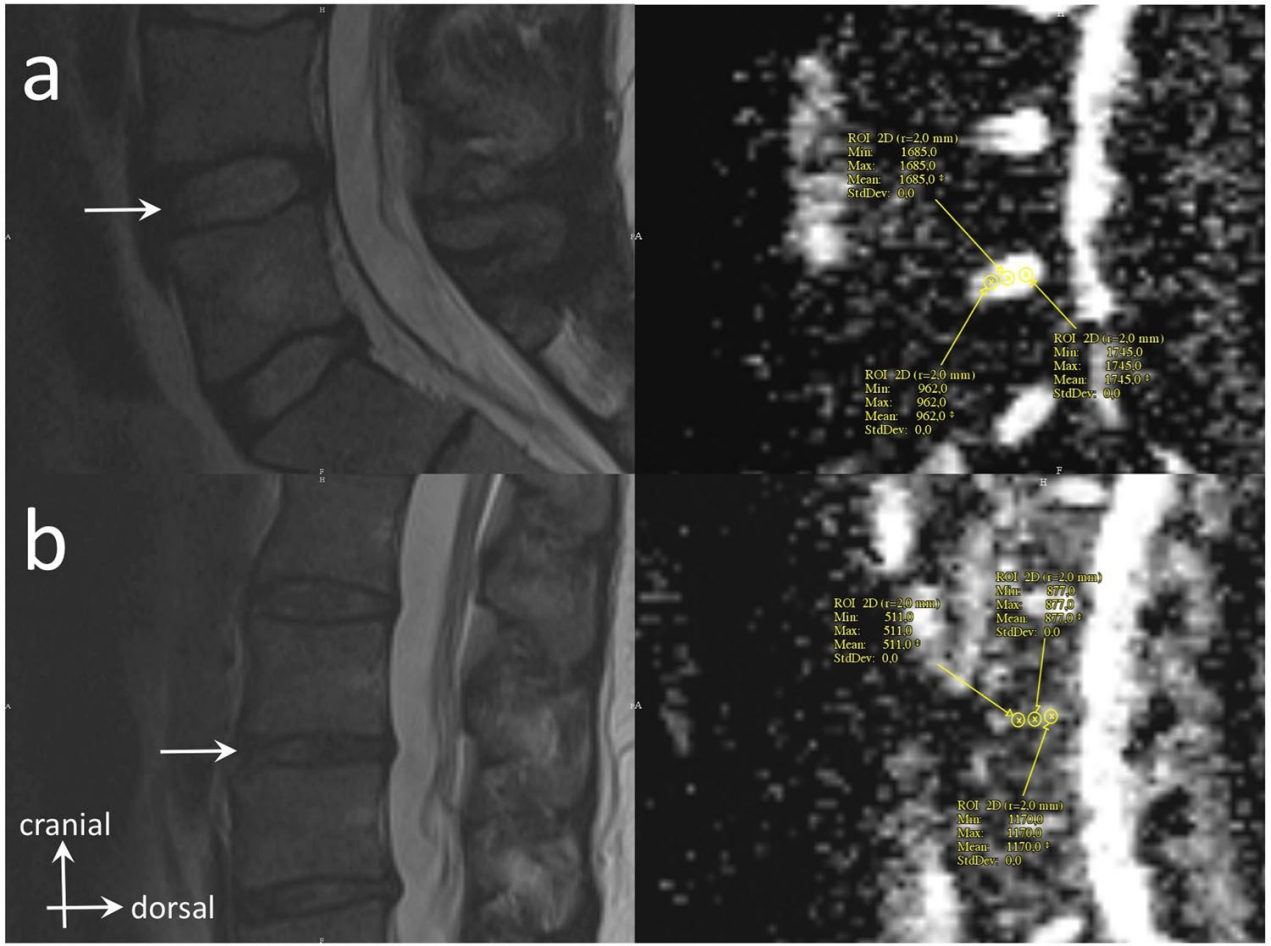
(a) T2-weighted image for an IVD classified as Pfirrmann’s grade 1 (arrow, left panel) and the corresponding diffusion-weighted image with the location of anterior, middle, and posterior ROIs used for the computation of $$ADC$$ values (right panel). (b) Similar T2- and diffusion-weighted images for an IVD classified as Pfirrmann’s grade 3.
